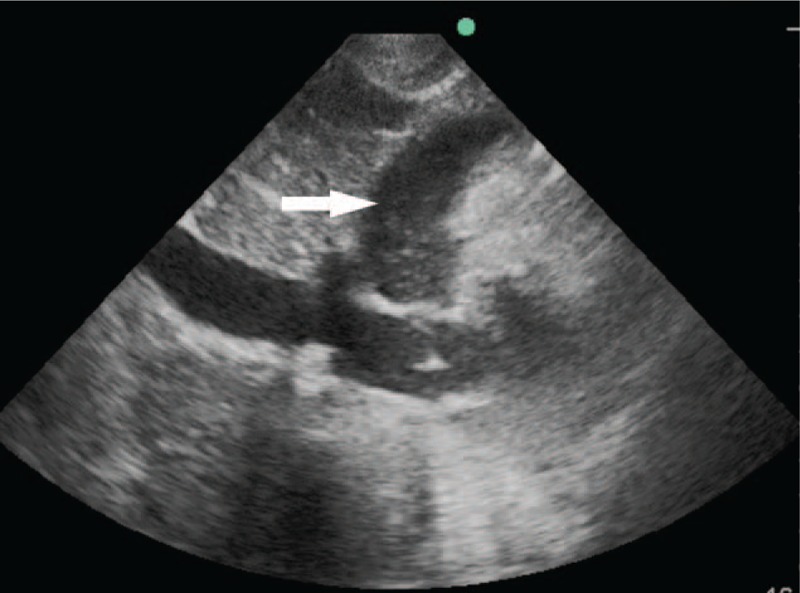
Bedside echocardiography revealed pericardial effusion.
